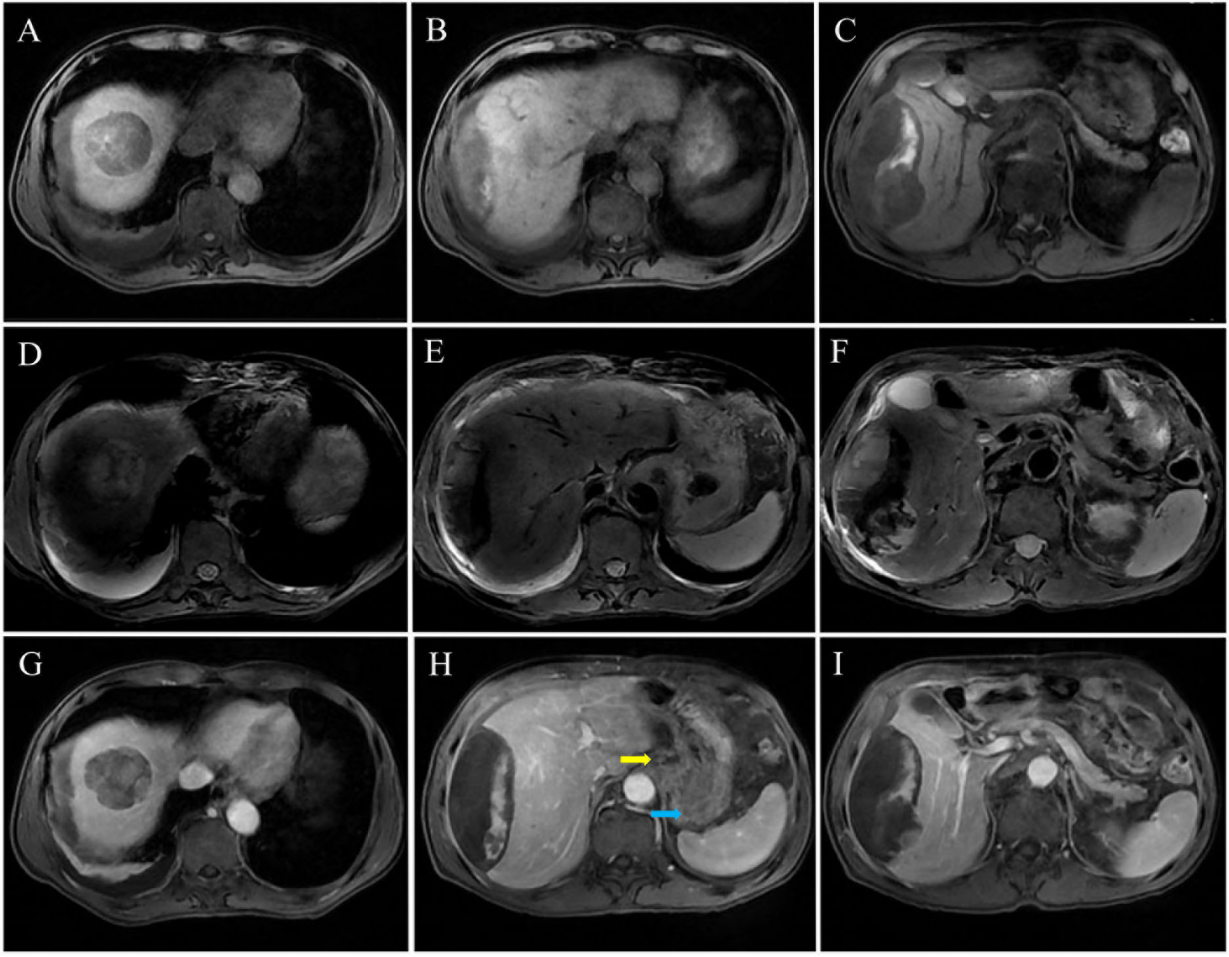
Contrast-enhanced MRI images of the GHA patient. (A-C) On T1-weighted imaging, hepatic metastatic adenocarcinoma typically presents as hypointense nodules or masses with signal intensity lower than that of the surrounding normal liver parenchyma. (D-F) On T2-weighted imaging, hepatic metastatic adenocarcinoma usually appears as hyperintense nodules or masses with signal intensity higher than that of the surrounding normal liver parenchyma. (G-I) MRI findings in the delayed phase showing a typical “bull’s-eye sign”. (H) Enlarged lymph nodes adjacent to the gastric antrum (the yellow arrow), with significant thickening of the gastric wall (the blue arrow).
